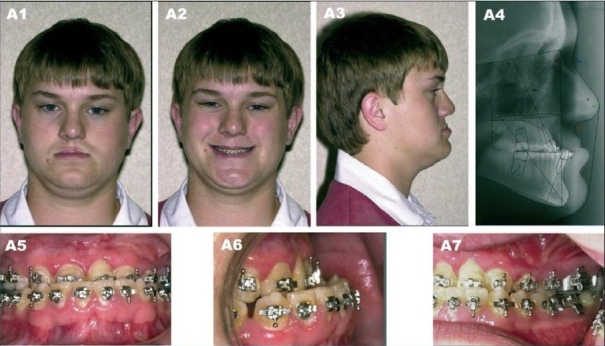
(A) A 16 year-old patient with unilateral complete cleft lip/nose, alveolus and palate after completion of growth and before orthognathic surgery. (A1) Frontal view (A2) Frontal smiling (A3) Right lateral view (A4) Preoperative lateral cephalometric roentgenogram (A5) Frontal view with orthodontic brackets (A6) Right oblique view with orthodontic brackets (A7) Left oblique view with orthodontic brackets
